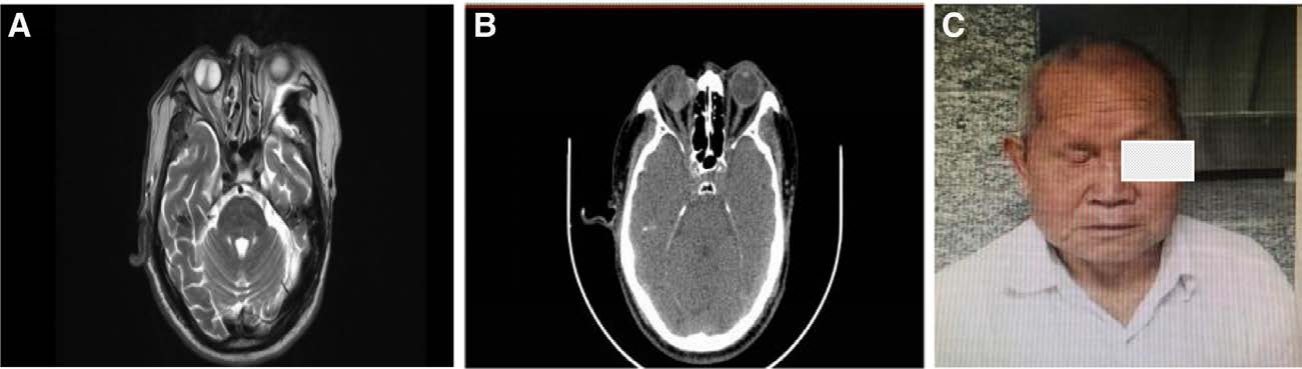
MRI (A): It demonstrated soft tissue thickening but no obvious enhancement in the right upper eyelid and lacrimal sac area. CT scan (B): It demonstrated the thickened soft tissue of the right eyelid was obviously alleviated than that before. (C) The patient after 2 years follow-up. CT = computed tomography, MRI = magnetic resonance imaging.
